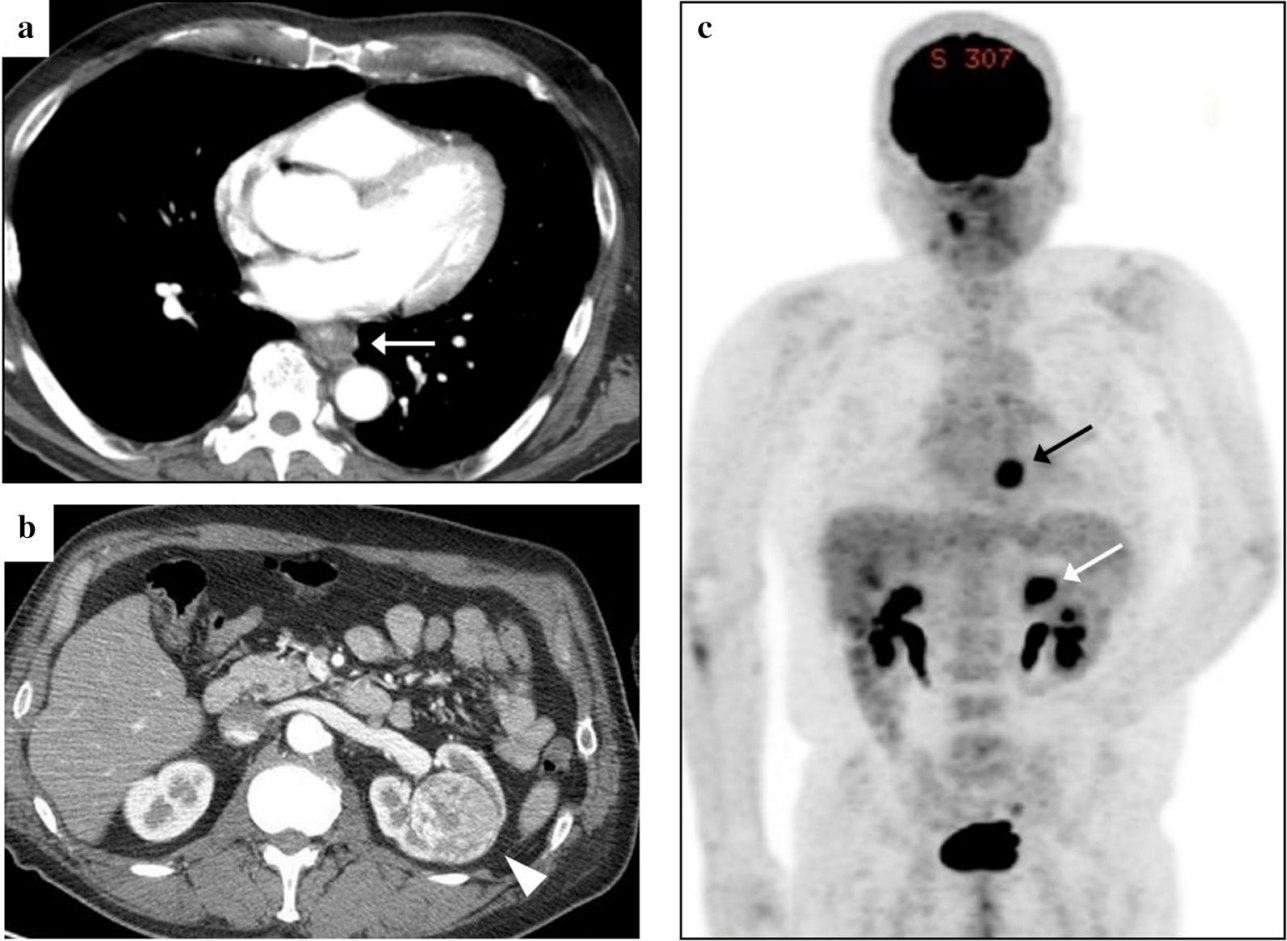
Enhanced CT examination showed a tumorous lesion occupying the esophageal lumen in the lower thoracic esophagus (arrow) (a). A 48-mm-diameter hypervascular tumor in the left kidney medulla (arrowhead) (b). High accumulation of 2-[^18^F] fluoro-2-deoxy-d-glucose (FDG) was observed in esophageal lesion and left kidney lesion (black and white arrow, respectively). There was no FDG accumulation indicating lymph node and distant organ metastasis (c)
